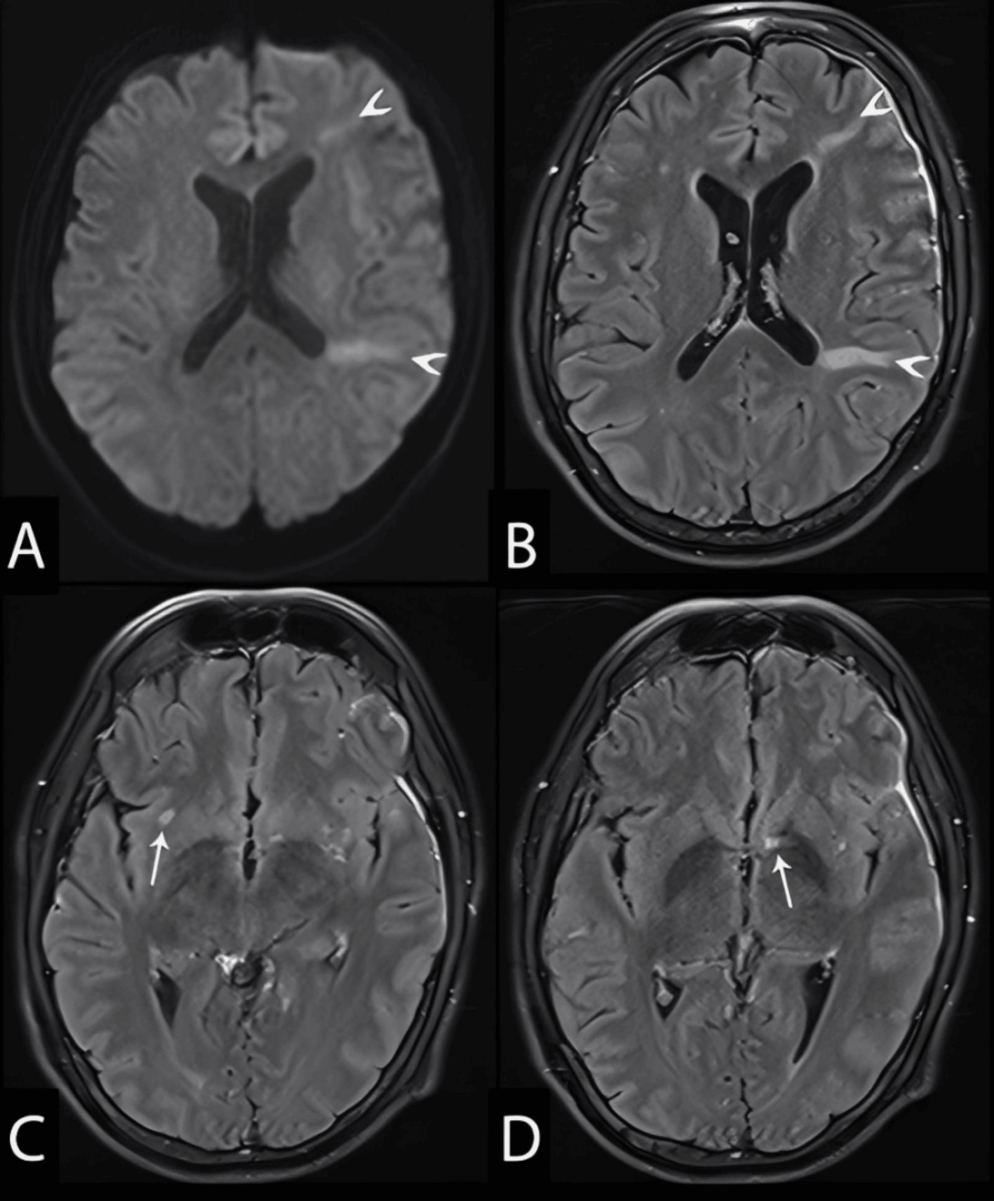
Brain MRI on day 65 Axial images. Panel A, DWI b = 1000; panels B-D, post-contrast T2 FLAIR images. New subacute ischemic infarctions are seen in the left frontal and left parietal corona radiata (arrowheads). Additionally, two focal ischemic infarcts have developed since the prior study: one in the right external capsule (arrow, panel C) and one in the left caudate head (arrow, panel D). Persistent leptomeningeal thickening along the left frontal lobe is similar to the previous study (B-D). DWI, diffusion-weighted imaging; FLAIR, fluid-attenuated inversion recovery
